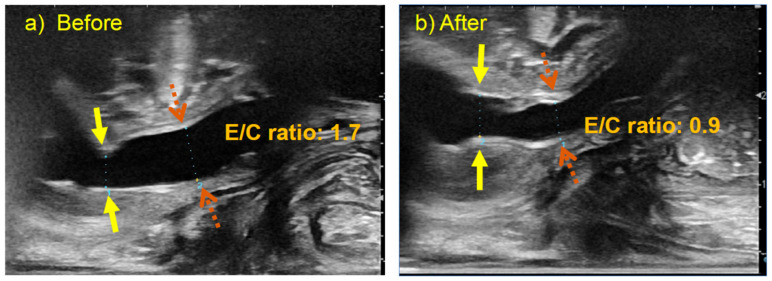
Comparison of urethral ultrasound images before and after urethral incision in a 2-year-old with PUV. Yellow solid arrows indicate the constricted (C) portion, and brown dashed arrows indicate the expanded (E) portion of the urethra. (a) Ultrasound finding before the urethral incision. The posterior urethra is dilated because of PUV. Urethral diameter at the (C) and (E) were 3.3 mm, 5.7 mm, E/C ratio: 1.7. (b) Ultrasound finding after the urethral incision. The narrow portion of the urethra due to PUV is relieved. Urethral diameter at the (C) and (E) were 4.7 mm, 4.1 mm, E/C ratio: 0.9. PUV: posterior urethral valves, E/C ratio: ratio of the diameters of the constricted (C) and expanded (E) portions of the urethra.
